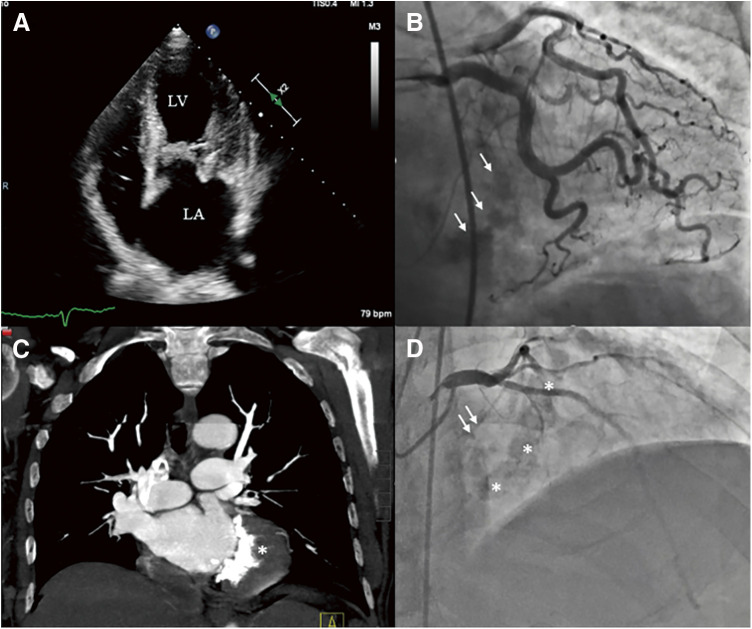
Preoperative imaging studies of different patients in which the current technique was successfully performed showing (A) echocardiogram with severely thickened native mitral valve and posterior annular calcifications, (B) preoperative coronary angiogram showing the posterior mitral annular calcification (multiple white arrows), (C) preoperative computed tomography scan showing severe mitral annular calcification (asterisk), and (D) preoperative coronary angiogram showing moderate degree of posterior mitral annular calcification (multiple asterisks) with minimal anterior calcifications (two white arrows). LA: left atrium; LV: left ventricle
